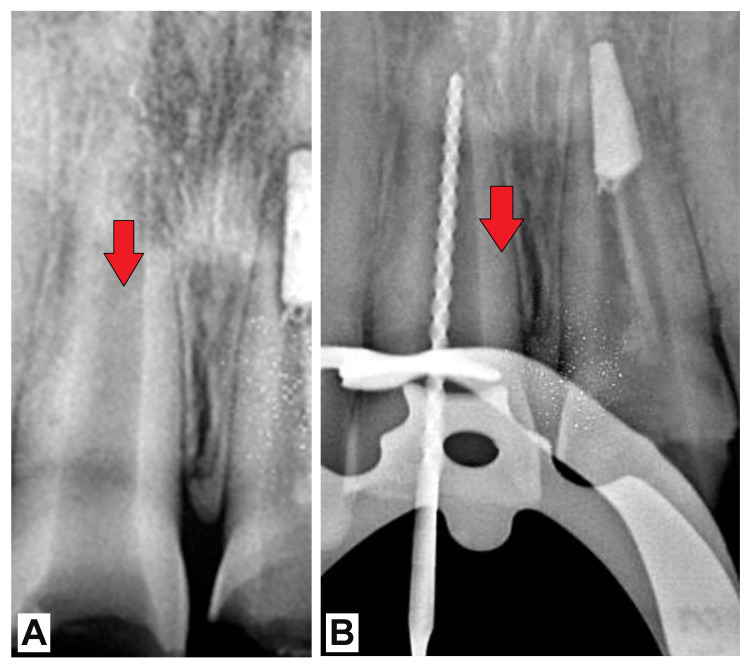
(A) Preoperative radiograph of the patient and (B) working length (red arrows)
